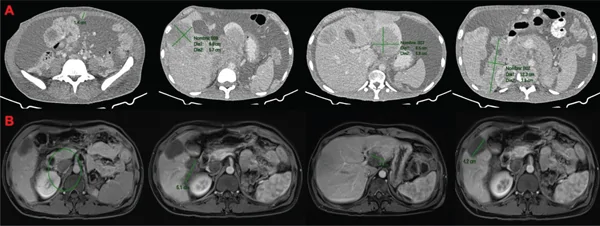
(A) Axial contrast‐enhanced computed tomography (CT) images from April 2024 demonstrate multiple intra‐abdominal metastatic lesions, including peritoneal implants, retroperitoneal lymphadenopathy, and liver metastases. (B) Axial magnetic resonance imaging (MRI) after systemic therapy with pembrolizumab plus lenvatinib shows marked reduction in lesion size across all metastatic sites, suggesting a significant partial response per RECIST v1.1.
